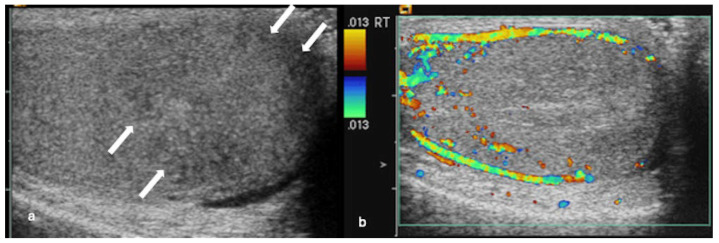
Segmental infarction. Longitudinal images of the left testis: (a) grey-scale ultrasound shows an ill-defined hypoechoic intratesticular lesion (arrows); (b) colour Doppler ultrasound demonstrates no detectable intralesional vascularity.
